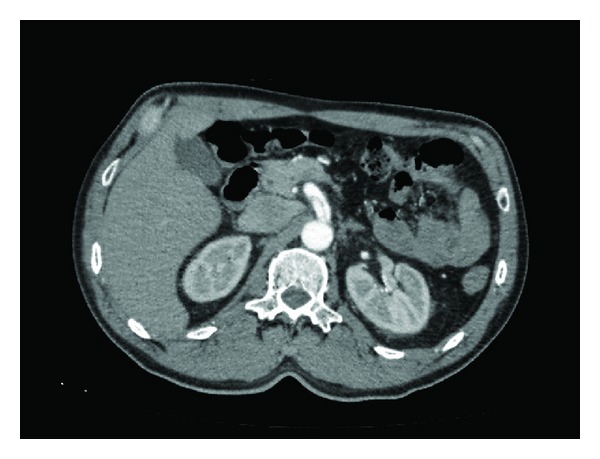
Computed tomography angiogram (axial view) revealing the double-barrel sign of the dissected superior mesenteric artery.
